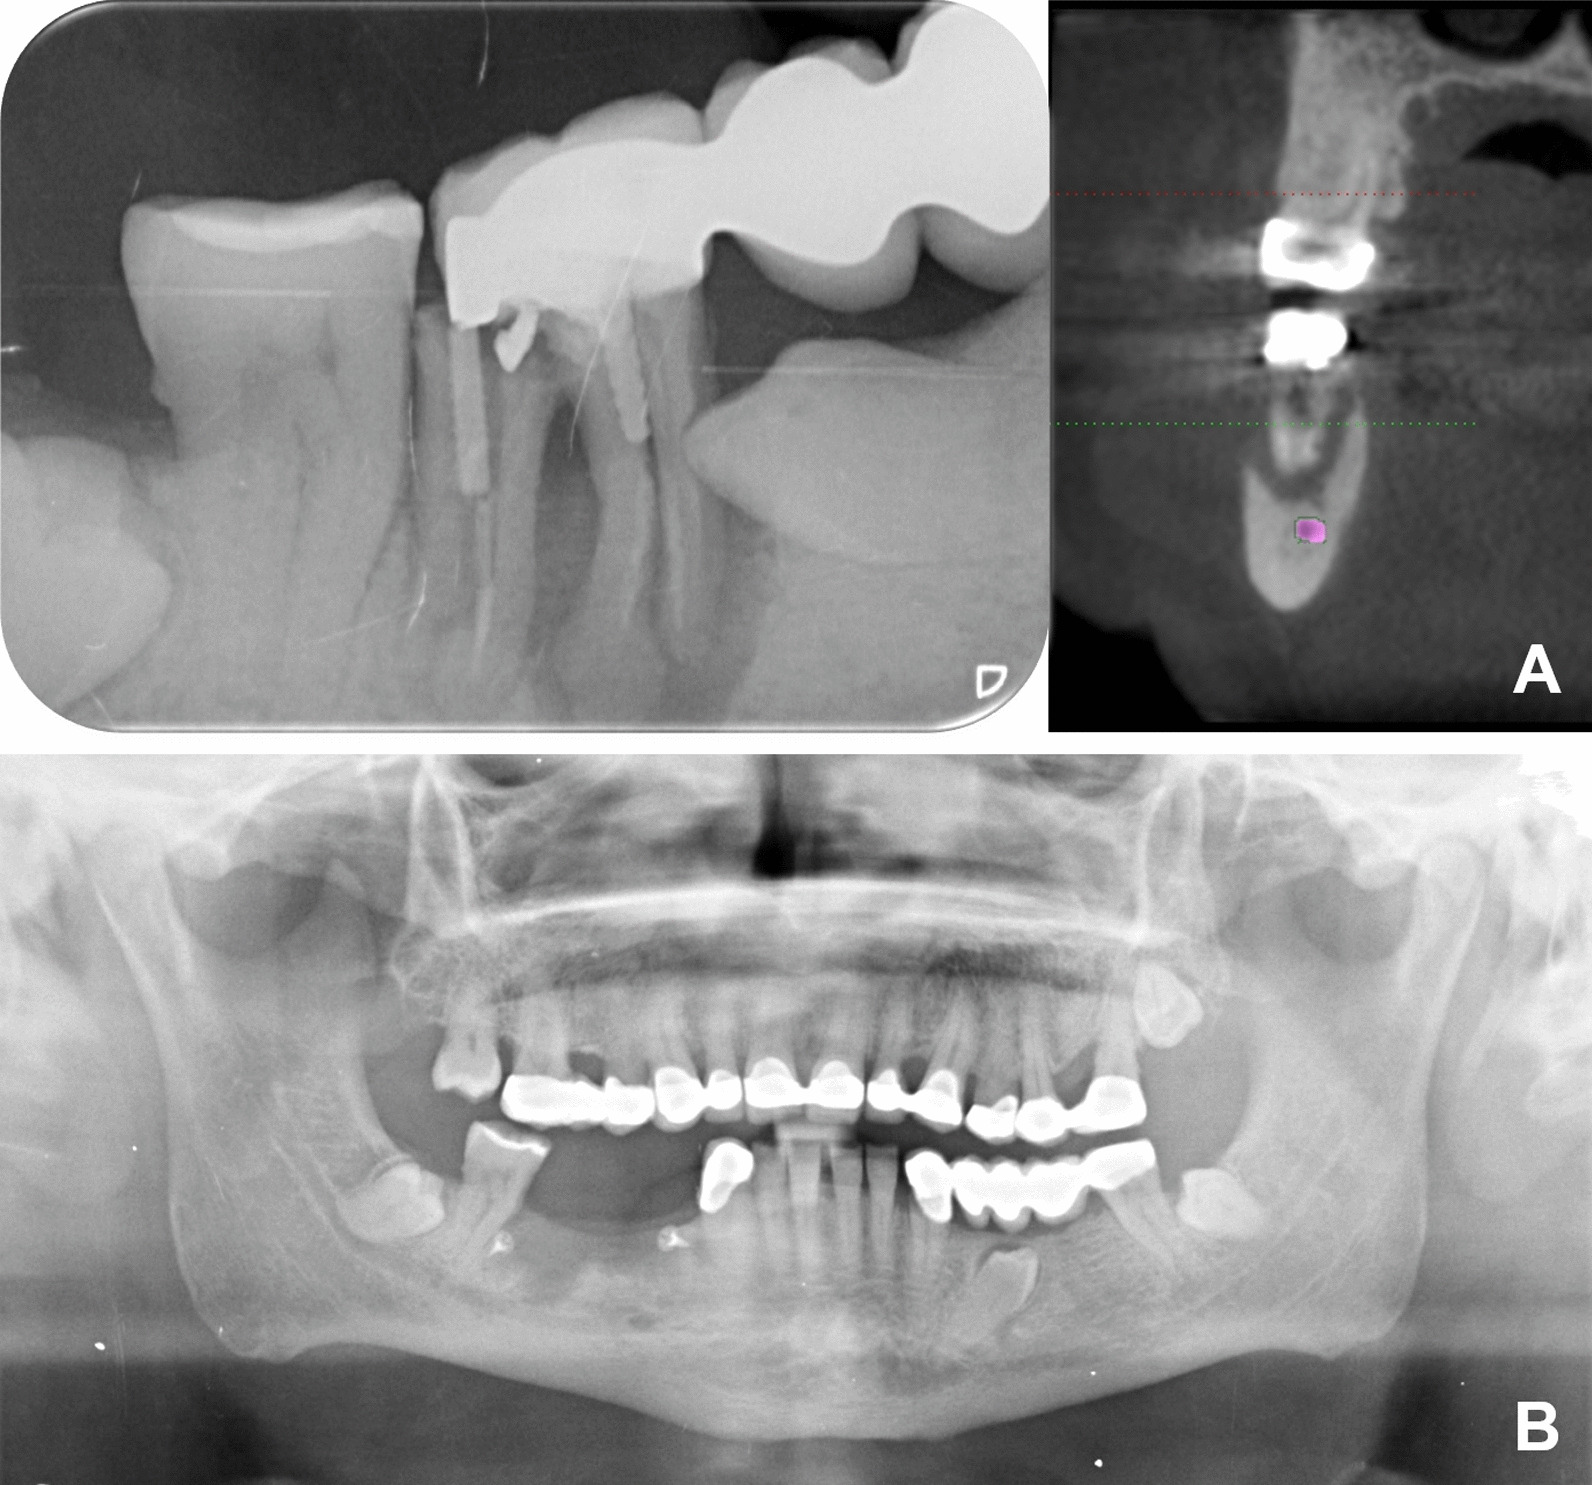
A Initial radiographical aspect: Radiolucent endo-periodontal lesion showing fracture of 4.6 with hypercementosis, inclusion of 4.5, and alveolar bone loss. B Presurgical radiographical aspect: Irregular radiolucent lesion in the previously operated area
